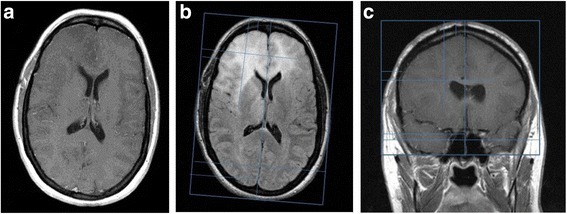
a, b & c demonstrate treatment planning in a patient with exclusively nonmeasurable disease: Figure a is the axial T1 post-contrast slice demonstrating no contrast enhancement. Figure b is the corresponding axial FLAIR sequence was used to map the tumor coordinates. A reference frame is drawn around the level of the scalp and tumor location coordinates are obtained from the right framebased on the extent of abnormal T2 signal. Figure c depicts the coronal treatment planning utilizing the T1 post-contrast sequence. The area of FLAIR signal abnormality corresponds with an area of T1 hypointensity on the coronal sequence. Treatment is planned to the boundaries of the T1 hypointensity at the treating physician’s discretion. Tumor location coordinates are obtained in a standard fashion from a reference drawn at the level of the scalp. The reference frame encompasses all of the supratentorial brain so the lower boundary is extended to the inferior margin of temporal lobe in this slice
